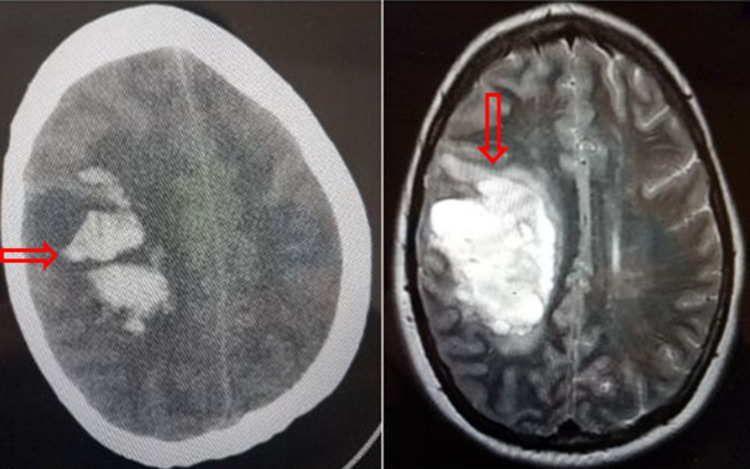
CT scan of the head (left) and MRI of the brain (right), both showing large multifocal intraparenchymal hemorrhage (red arrows) of the right frontal and parietal lobe with associated edema and mass effect causing subfalcine herniation and rightward transtentorial herniation CT: computed tomography; MRI: magnetic resonance imaging
